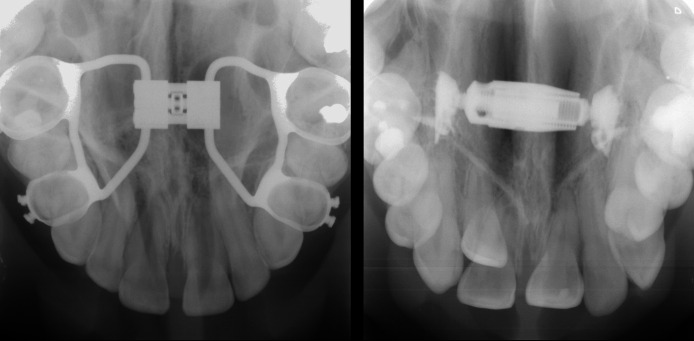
Occlusal radiographs of an individual Hyrax appliance (left) and a standardised transpalatal distractor (right). Radiographs were taken after surgery and before postoperative activation
